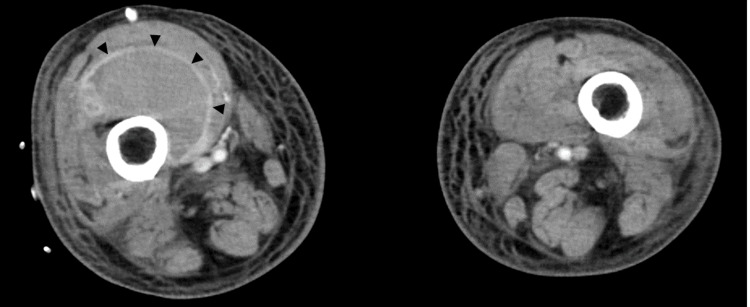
CT findings of an intramuscular abscess The patient presented with pain in the right thigh, and a CT scan revealed an intramuscular abscess in the same region (triangle marker).
